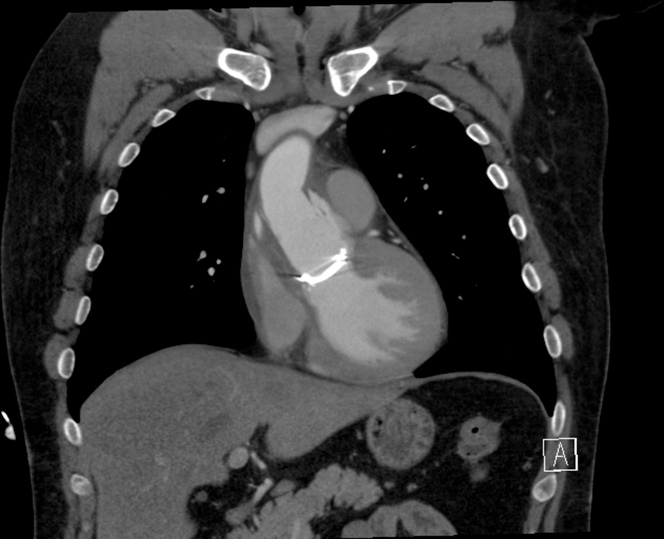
Coronal Computed Tomography Chest View Demonstrating Pseudoaneurysm of the Sinuses of Valsalva Coronal view of the computed tomography of the chest demonstrating contour abnormality involving the sinuses of Valsalva extending from the aortic root consistent with pseudoaneurysm.
